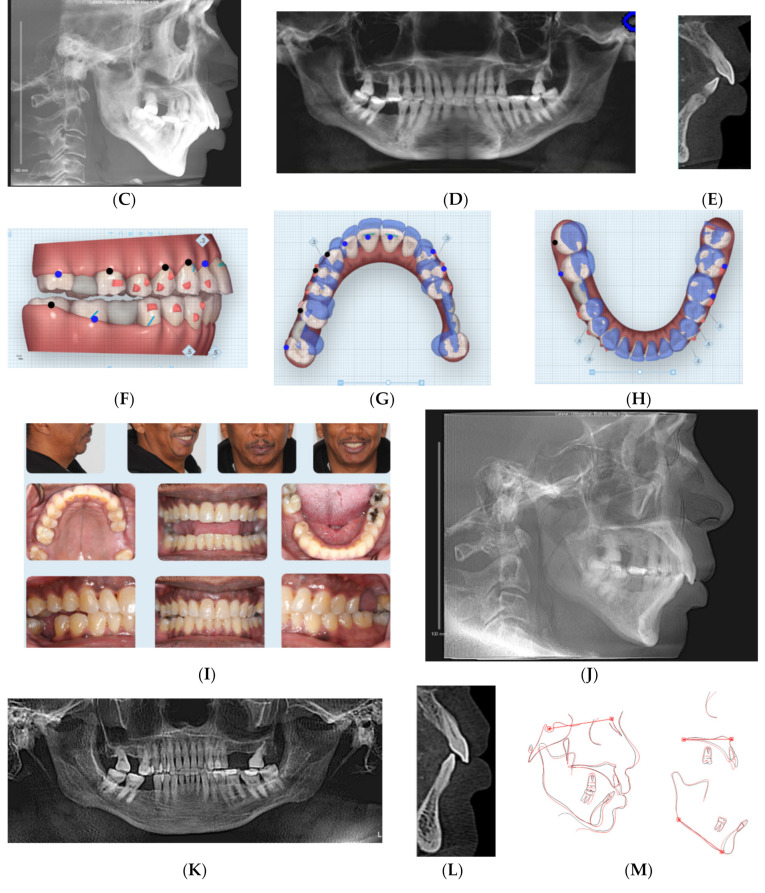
(A) Initial photographs showing convex profile with recessive chin and anterior crowding. (B) Initial digital models showing left side Class II buccal occlusion with a 7 mm increased overjet. (C) CBCT-driven cephalometric radiograph showing convex profile with recessive chin and protrusive upper incisors. (D) CBCT panoramic radiograph showing missing 1.7, 2.6, 2.7, and 4.6 molars; no other noticeable intraoral bony lesion could be detected. (E) CBCT-driven sagittal screen of lower incisor showing its severe proclination with minimum bone appears on most of the labial surface of the root and compromised crown/root ratios. (F) Digital treatment plan (ClinCheck) showing posterior teeth intrusion to help forward rotation of the mandible palatal root torque of upper incisors. (G) Upper arch digital treatment plan (ClinCheck) showing expansion (blue—initial teeth position, white—final teeth position) and retraction of upper incisors to improve their initial protrusion. (H) Lower arch digital treatment plan (ClinCheck) showing (blue—initial teeth position, white—final teeth position) with interproximal reduction plan to allow lower incisor intrusion and leveling /alignment of lower incisor crowding. (I) Final photos showing improved patient’s profile and chin projection as well as aligned upper and lower incisors and Class I buccal occlusion on both sides. Additionally, some remaining posterior open bite due to the posterior teeth intrusion remains; the patient was happy and requested finishing by letting his posterior teeth settle through final retainers. (J) CBCT-driven final cephalometric radiograph showing improved profile and chin projection. (K) Final CBCT-driven panoramic radiograph. (L) Final CBCT-driven sagittal screen radiograph of upper and lower incisors showing improved overjet and bone labial to both upper and lower incisors. (M) Superimposition of cephalometric tracing of before (black) and after (red) treatment showing improved forward chin projection, palatal movement of upper incisors, and profile and chin projection and lingual tipping of lower incisors.
